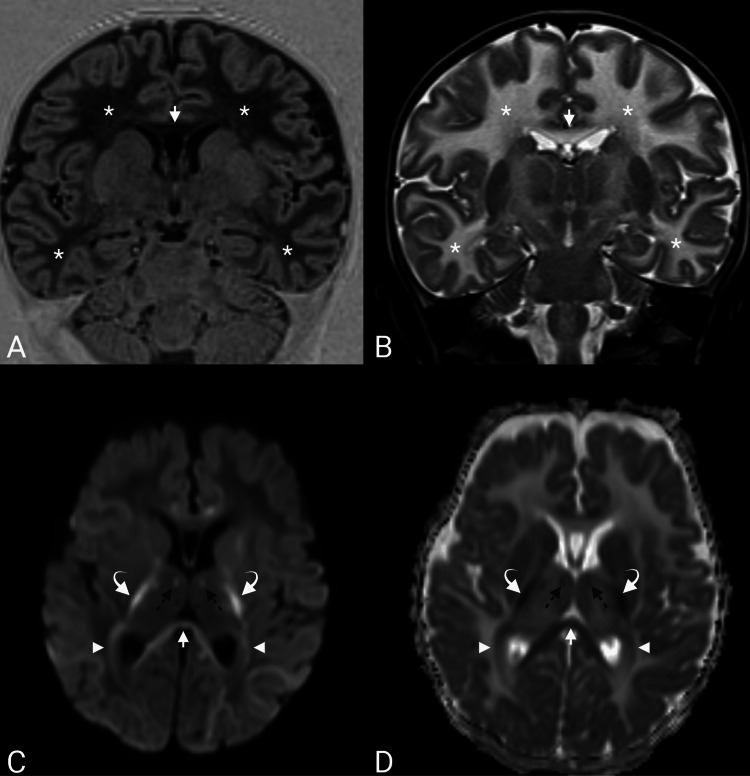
Diffuse dysmyelination with superimposed diffusion restriction. (A) Coronal T1 inversion recovery and (B) T2-weighted images show diffuse T1 hypointensity and T2 hyperintensity involving the deep and subcortical white matter (white asterisks) and the corpus callosum (white arrows). (C) Diffusion-weighted imaging and the corresponding (D) apparent diffusion coefficient map demonstrate restricted diffusion involving the internal capsules (curved white arrows), corpus callosum (white arrows), mammillothalamic tracts (black dotted arrows), and optic radiations (white arrowheads).
